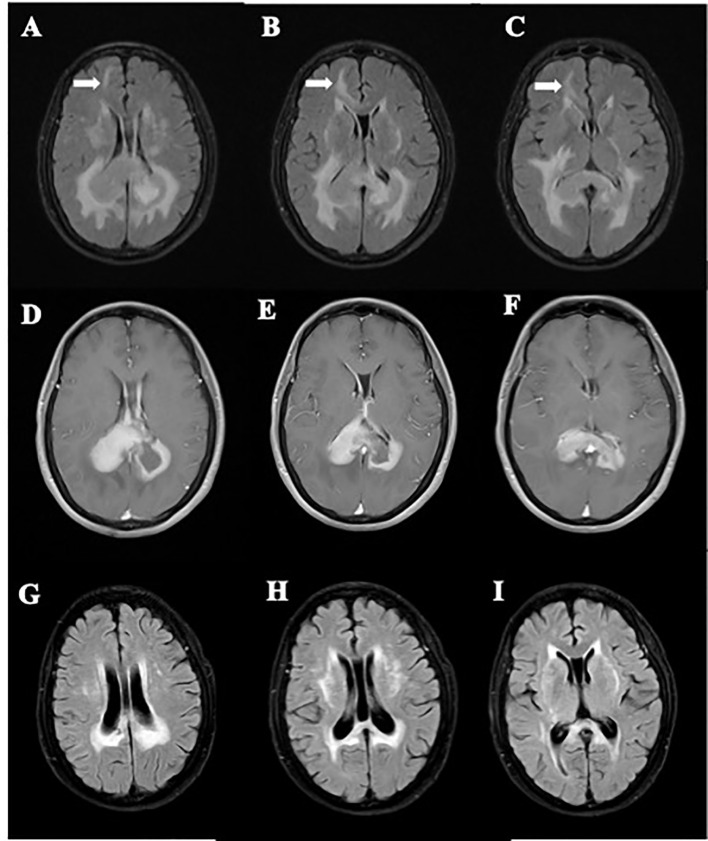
MRI findings in a 51-year-old woman. (A–C) T2-FLAIR images show linear high-signal lesions in the deep white matter of the right frontal lobe (white arrow). (D–F) These lesions were not enhanced on axial T1-weighted images with contrast enhancement. (G–I) After treatment during follow-up, axial T2-FLAIR images revealed that the previous high-signal lesions had markedly decreased.
